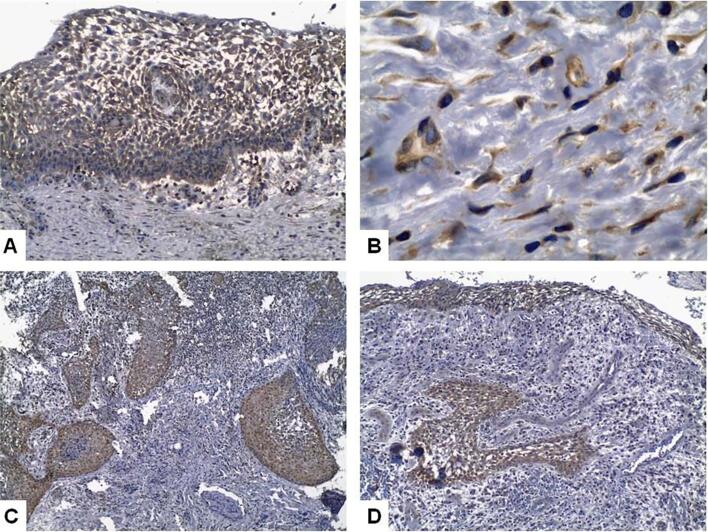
KGF expression in the hyperplastic epithelial lining (A, Immunoperoxidase 200x) and in fibroblasts in the fibrous capsule of a periapical cyst (B, Immunoperoxidase 1000x); EGFR expression in proliferating odontogenic epithelium in the capsule of a periapical cyst (C, Immunoperoxidase 100x), and in the epithelial lining and in the proliferating epithelial rest in the capsule of a periapical cyst (D, Immunoperoxidase 200x)
